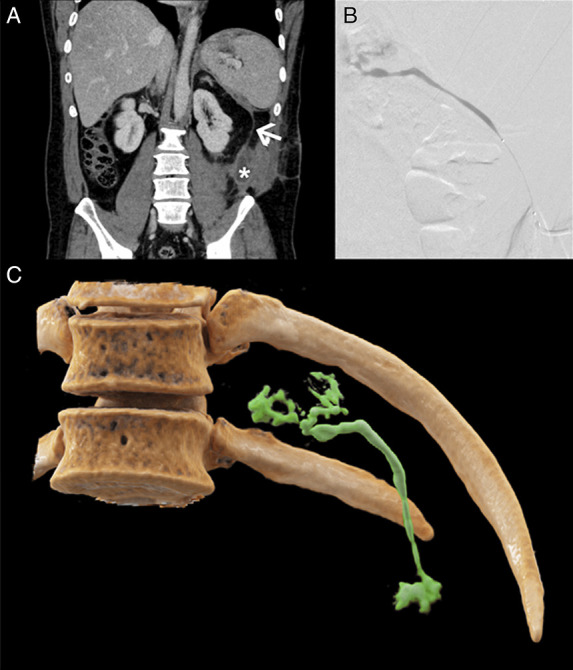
Computed tomography images of the patient depict subcutaneous fluid collection (indicated by asterisk [*]) caused by a pancreatic fistula in the area of the former percutaneous drainage (as shown by the arrowhead) (A). During the intervention, complete contrasting of the formation was achieved after injection of contrast medium and a 1:4 mixture of Histoacryl and lipiodol were installed (B). A 3D-reconstruction of the postinterventional result is shown in (C).
